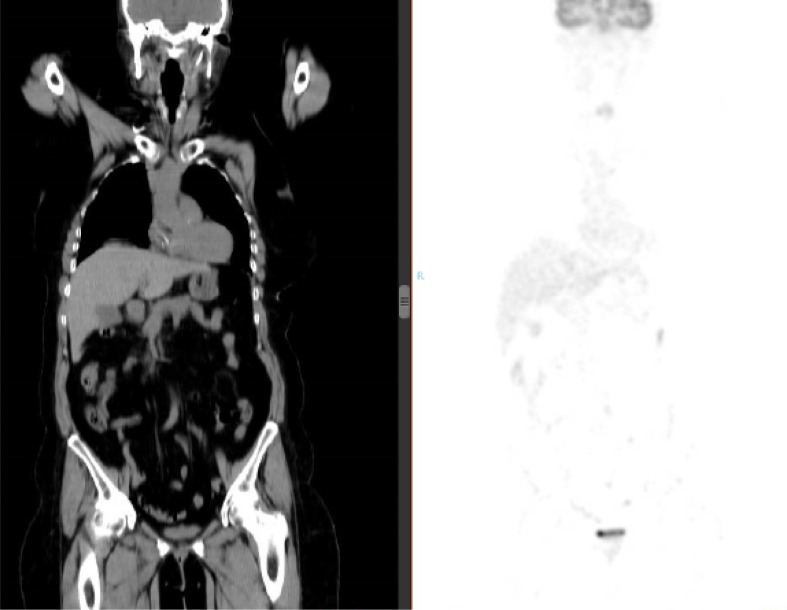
PET/CT showing no pathological uptake of 18-fluoro-deoxy-glucose. Complete biometabolic response is confirmed. Left of the figure is the corresponding CT image. Right of the figure is the image corresponding to the PET, with absence of pathologi-cal uptake
